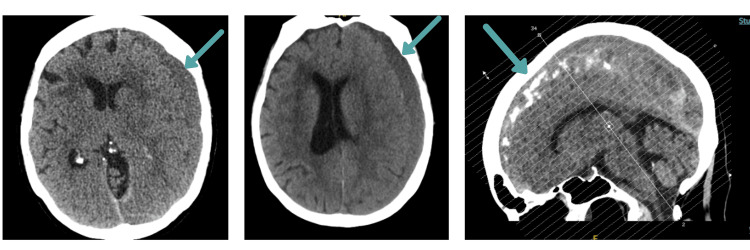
Follow-up CT head after inpatient fall CT demonstrating continued evolution of chronic subdural haematoma without acute rebleeding. Mass effect and midline shift remained stable compared to admission imaging.
